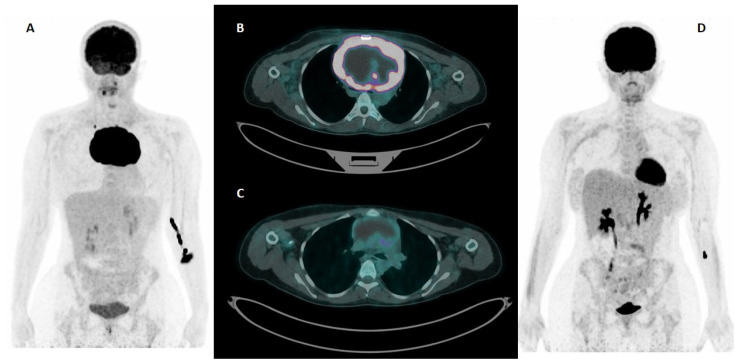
A case of PMBCL in a young woman (28 years old) from the University Hospital of Ferrara. Maximum intensity projection (A)—MIP—of baseline [^18^F]FDG PET/CT shows a large area of intense [^18^F]FDG uptake corresponding, in trans-axial fused PET/CT images (B), to a bulky mass of the anterior mediastinum. The image shows peripherical uptake with a “cold” core, corresponding to central necrosis. End-of-treatment [^18^F]FDG PET/CT shows a complete metabolic response (DS = 1) both in trans-axial fused PET/CT images (C) and at MIP (D). The patient did not experience disease relapse at follow-up (64 months).
